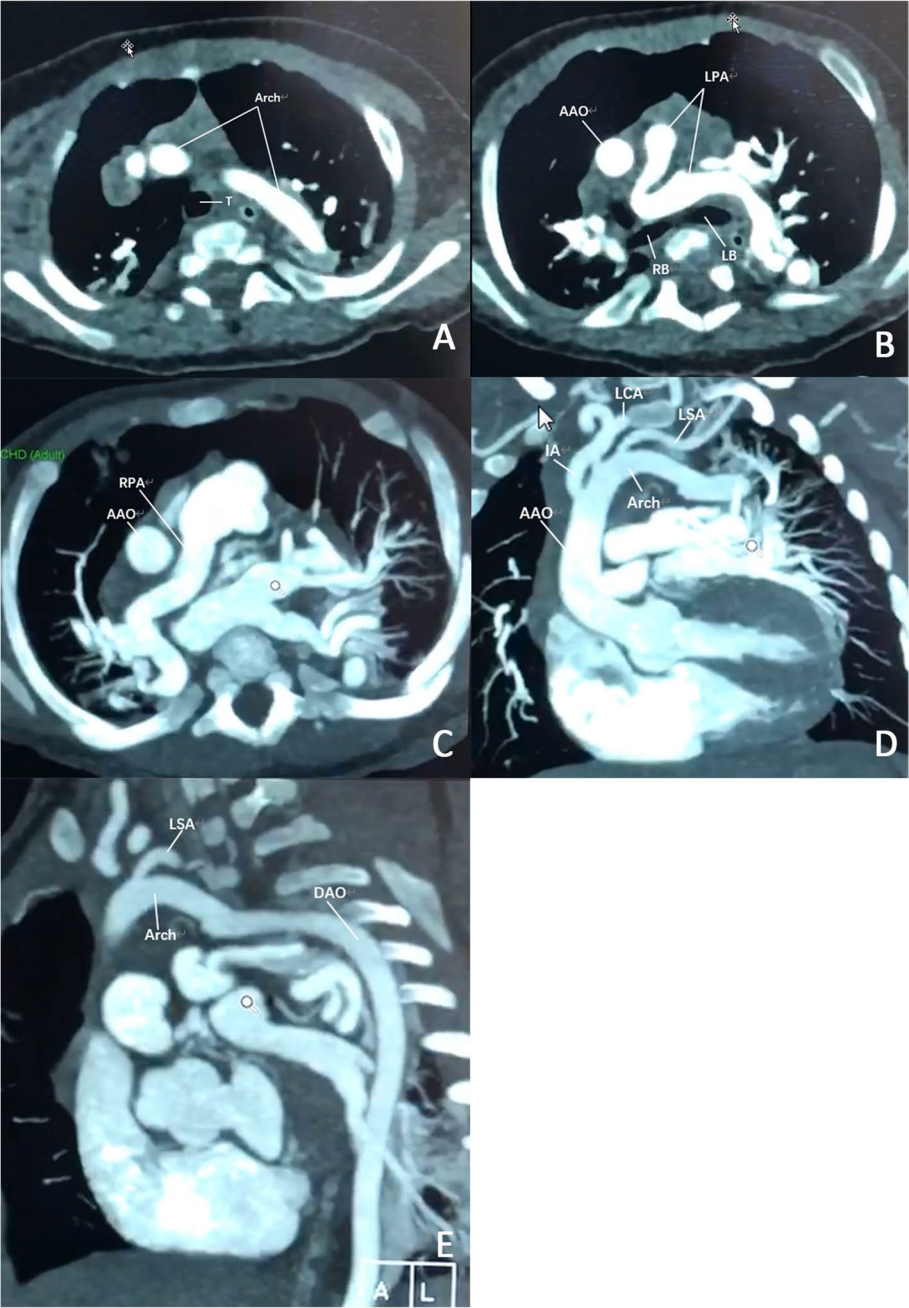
Contrast-enhanced computed tomography images of the older brother three months after delivery. A The CTA cross-sectional reveal the long aortic Arch in front of the trachea. B LPA are tortuous and dilated in front of the left and right bronchus. C RPA originated from MPA and also tortuous and dilated, the dot refers pulmonary vein. D Sagittal section of the aortic arch reveals three branches of Arch (IA, LCA, LSA) are tortuous. E The sagittal section of the descending aorta shows a very large vascular span of the DAO. Arch: aortic arch; AAO: ascending aorta; RPA: right pulmonary artery; LPA: left pulmonary artery; IA: innominate artery; LCA: left common carotid artery; LSA: left subclavian artery; DAO: descending aorta; T: trachea; LB: left bronchus; RB: right bronchus
